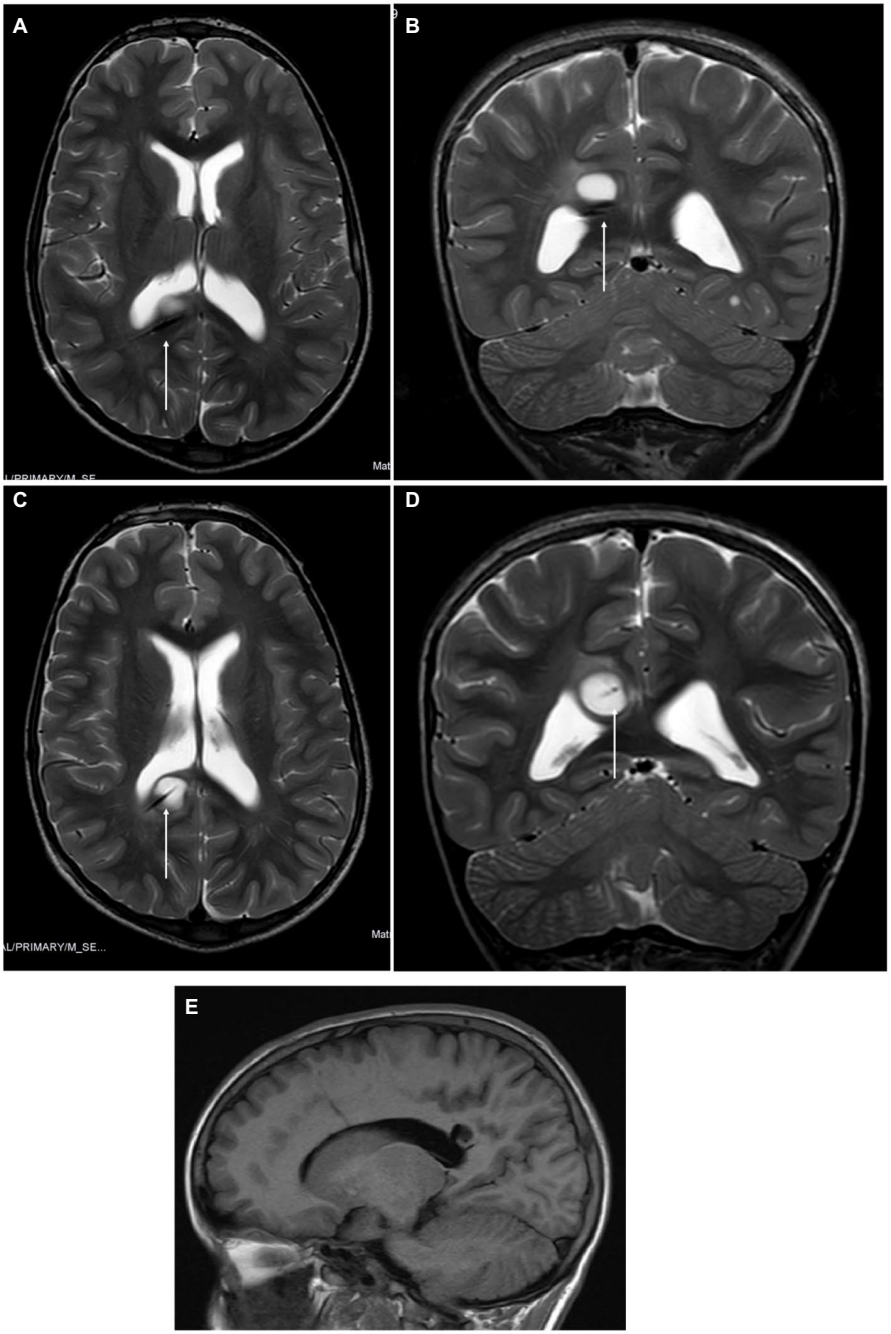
(A,B) Magnetic resonance imaging (MRI) revealed a suboptimal position of the tip of the laser diffusing fiber (LDF) on axial and coronal slices T2 sequences. No bleeding was detected. (C,D) MRI after repositioning the LDF before starting the procedure, with the tip on the middle of the right occipito-parietal tumor. (E) At the 21-month follow-up, the MRI scan revealed that the tumor had a cystic appearance and was filled with CSF. There was no evidence of contrast enhancement.
